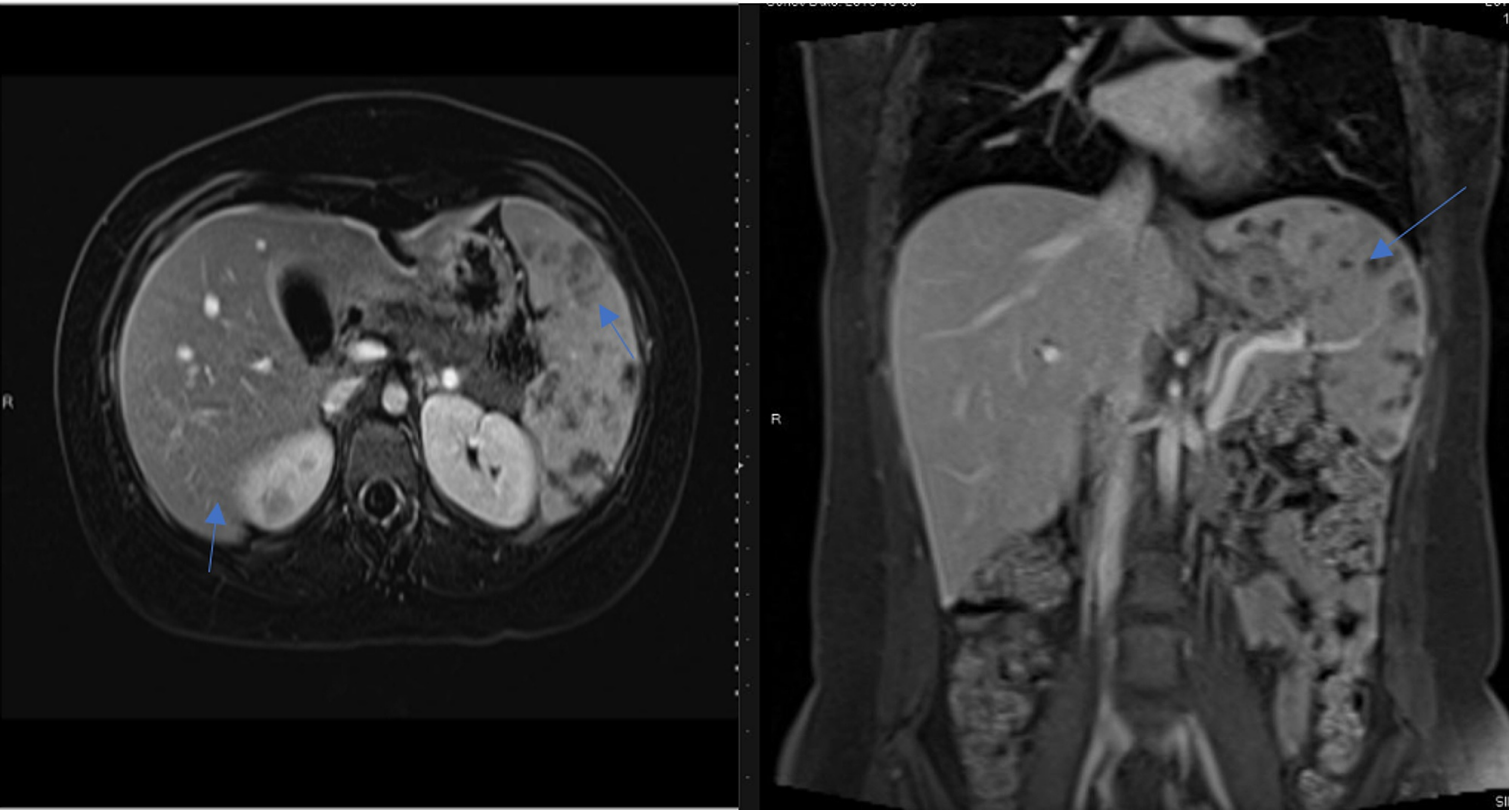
MRI of the abdomen - T1 weighted post-contrast transverse view (left image) and coronal view (right image) showing multiple hypodense lesions in the liver and spleen (marked by blue arrows)
